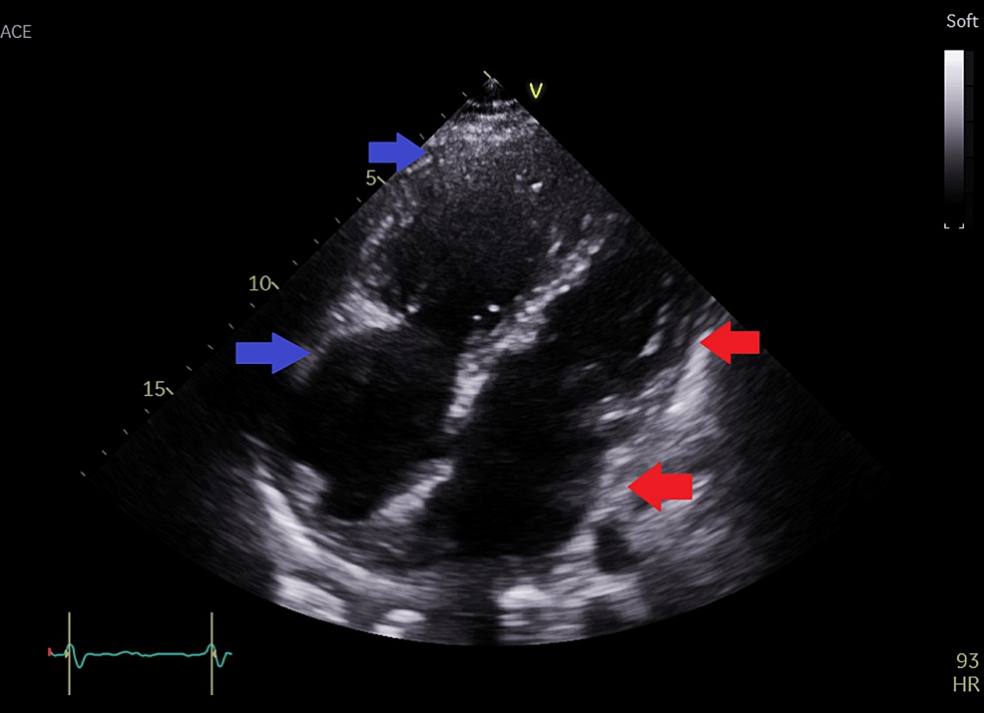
Modified apical four-chamber view showing dilated right atrium and right ventricle. blue arrows=right atrium and right ventricle; red arrows=left atrium and left ventricle
